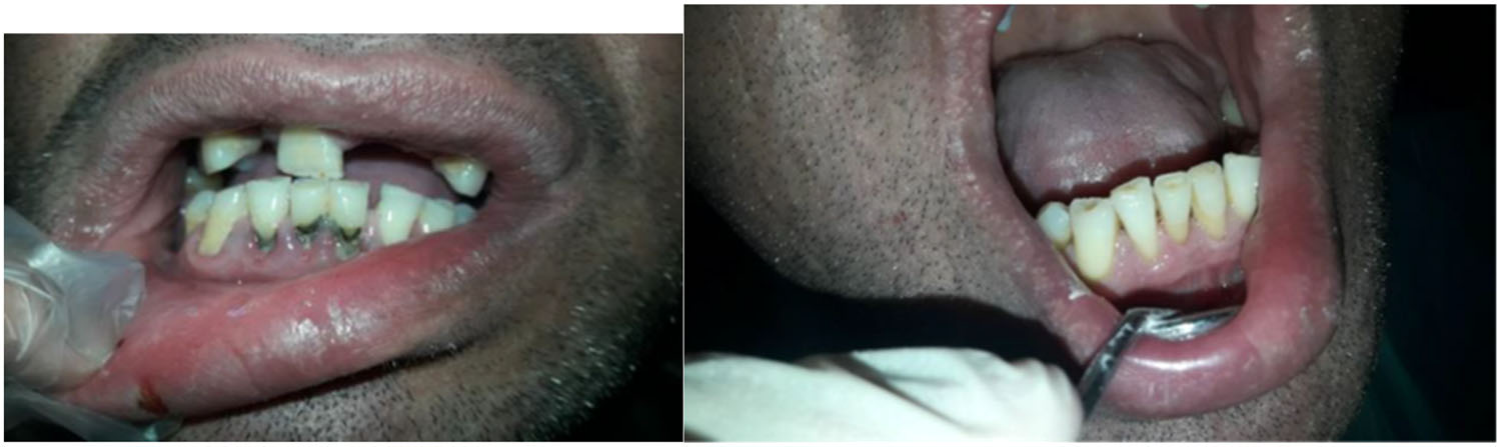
Before and after standard SRP treatment alone by the ultrasonic device.
